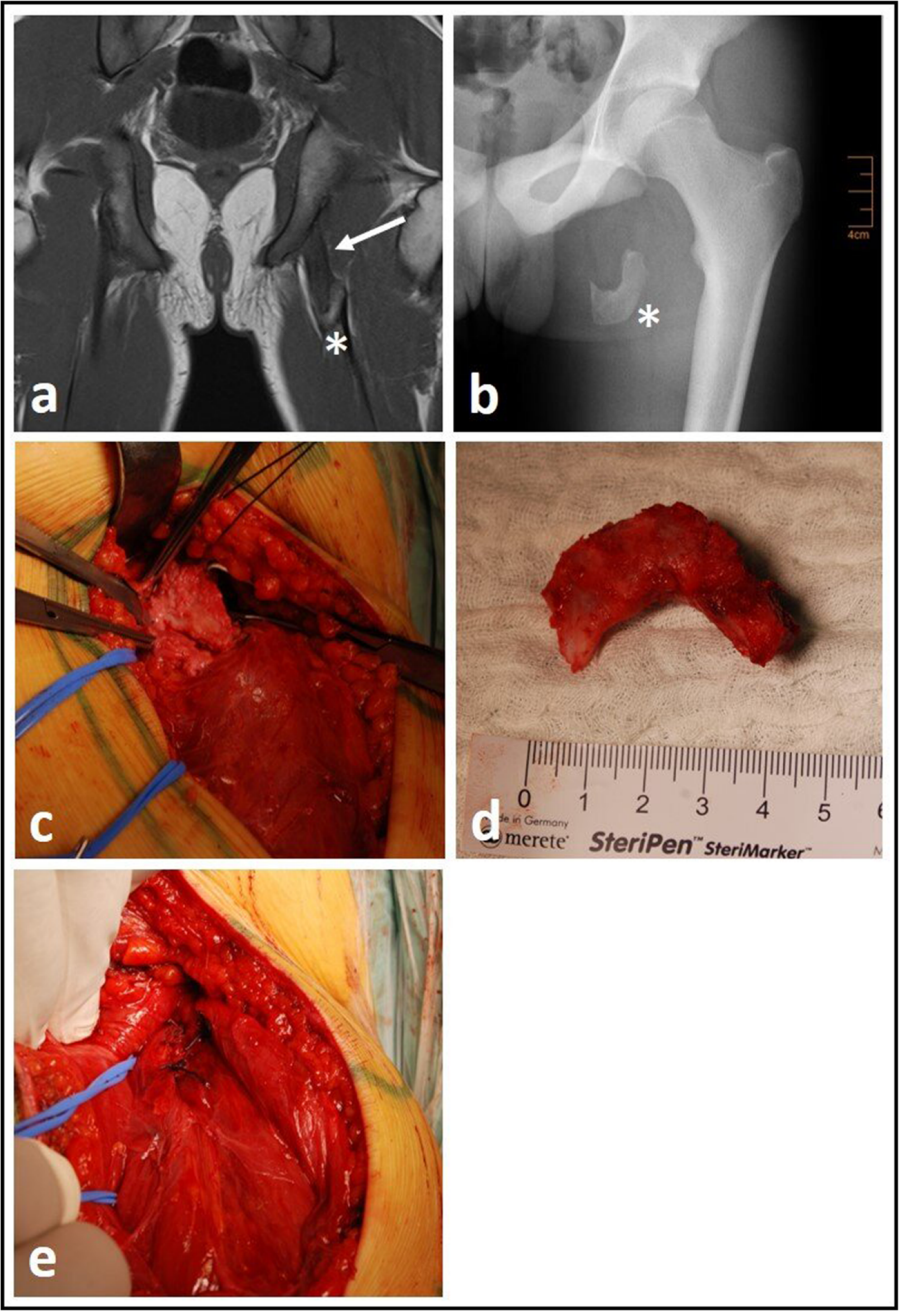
Excision of bony fragment in a chronic left-sided avulsion fracture one year after trauma (a: coronal MRI picture, b: plain semipelvis radiograph, c: intra-operatively dissected bony fragment, d: excised bony fragment, e: intra-operative end to end suture of tendinous scary plate, star*: bony fragment, arrow →: tendinous scary plate on MRI)
